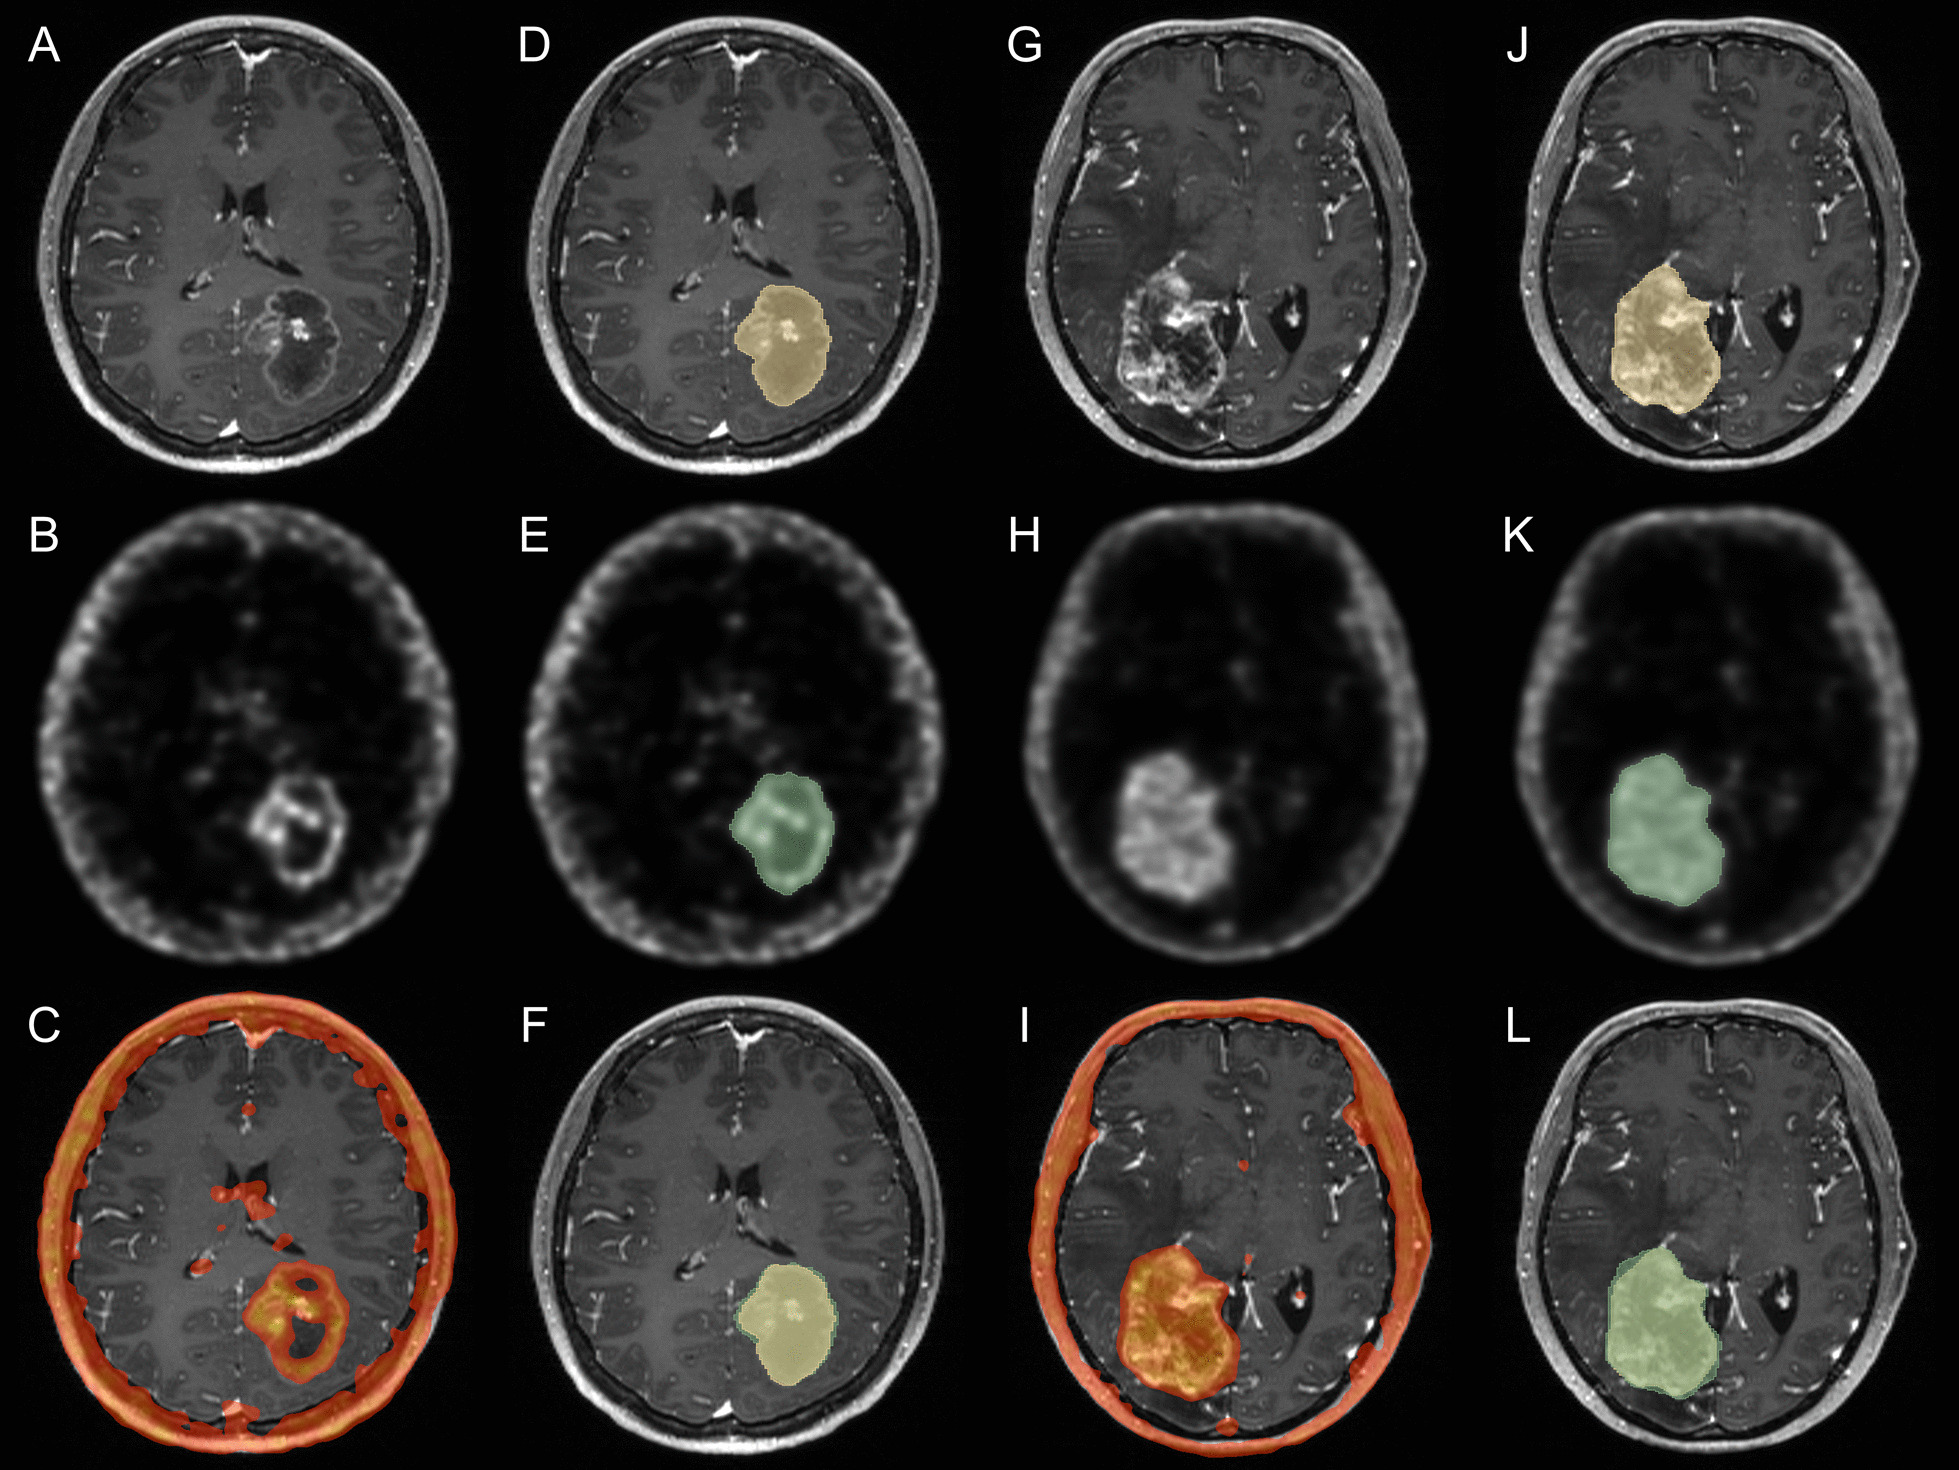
Examples of MRI and FBY PET images in circumscribed tumors. A left temporal-occipital metastatic breast cancer (HER2 positive, ER and PR negative) revealed significant contrast enhancement (A) and FBY activity (B), with better adhesion between the two regions (C). Semiautomated segmentation based on MRI (D) and FBY PET (E) was performed, and minimum FBY activity beyond MRI contrast enhancement was noted (F). Similarly, a right temporal-occipital metastatic breast cancer (HER2 negative, ER and PR positive) displayed contrast enhancement (G) and FBY uptake of the whole tumor region (H), both of which presented an explicit tumor extent (I). The semiautomated segmentations (J, K) were also largely equivalent (L), which may be due to the clear boundary of circumscribed tumors
